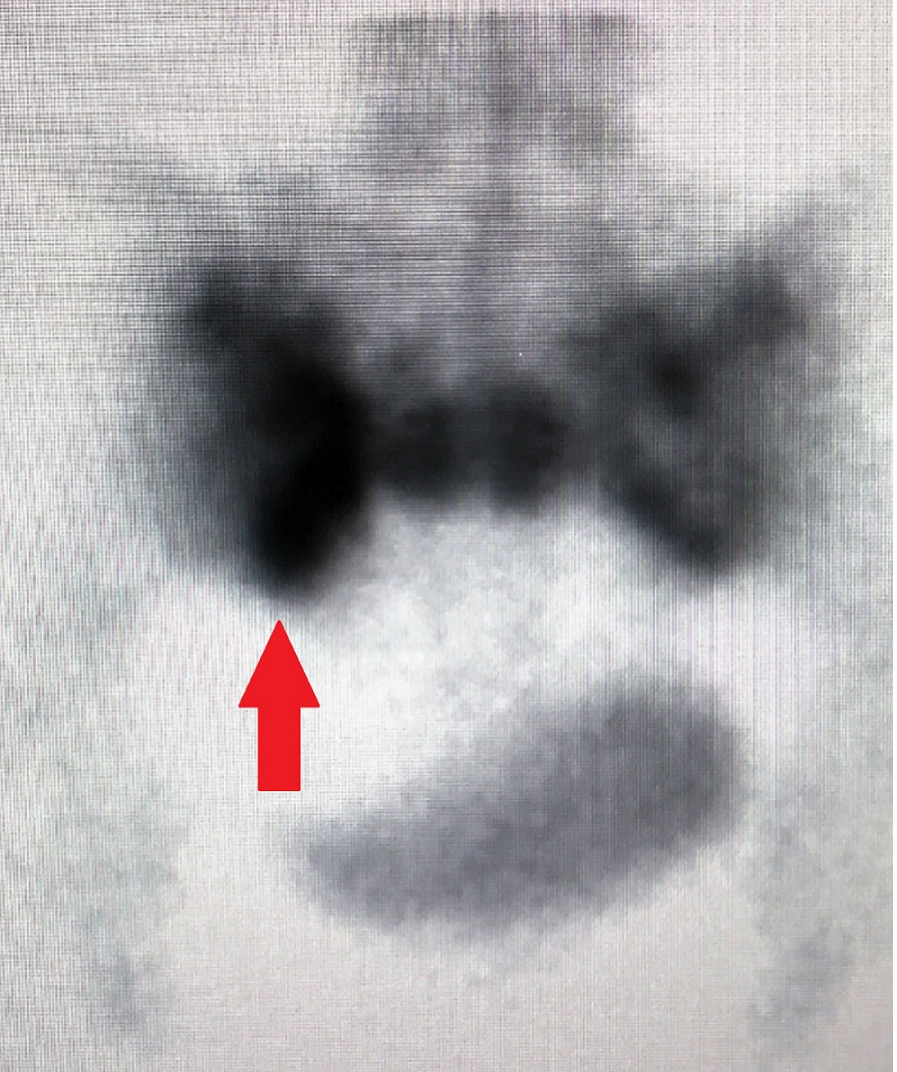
Technitium-99m labeled bone scintigraphy Increased uptake of technitium-99m, demonstrated by the arrow, in the bilateral sacral ala and across the body of the sacrum in the characteristic "Honda logo" shape
